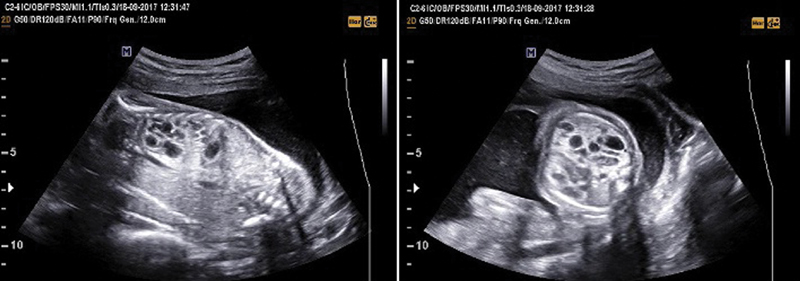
Prenatal ultrasonographic features of a CPAM case in our series.
